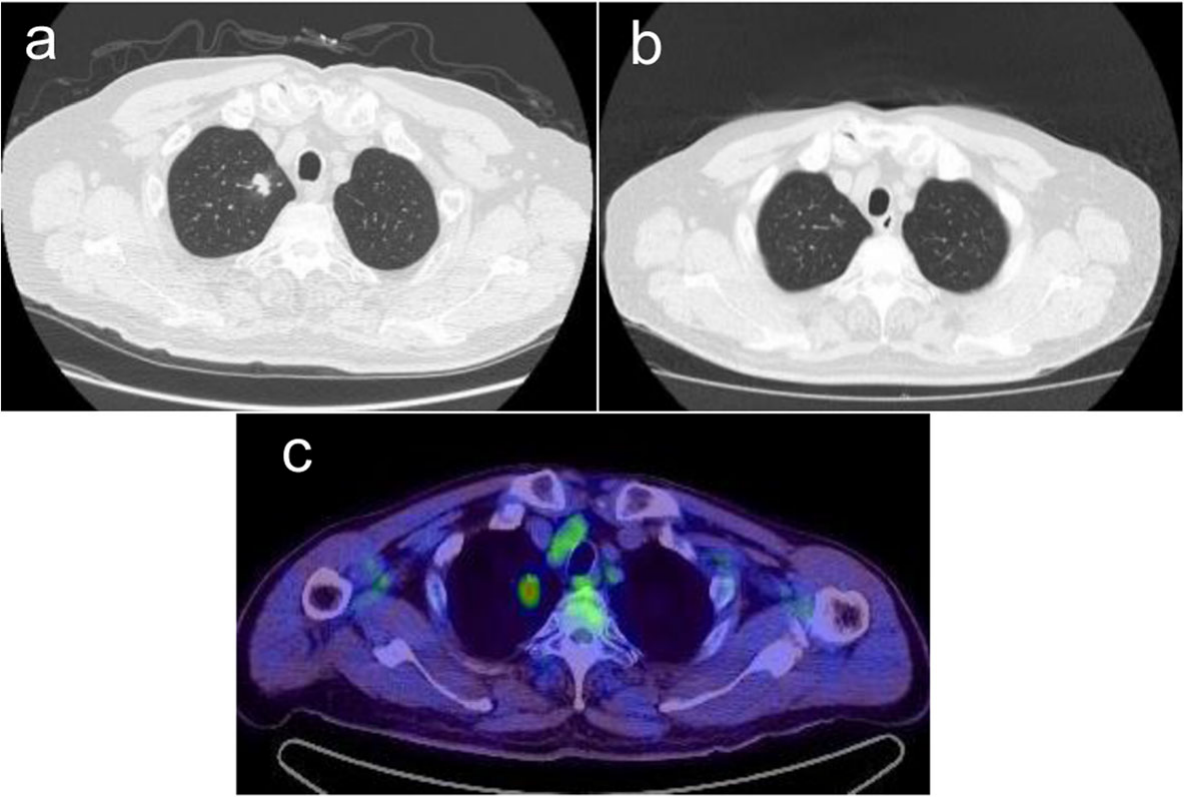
CT and PET/CT scans revealed a mass in the apex of the right lung. Chest CT showed a mass in the upper lobe of the right lung (a), which was not detected 2 months previously (b). In the PET/CT scan, this mass showed abnormal accumulation (c)
